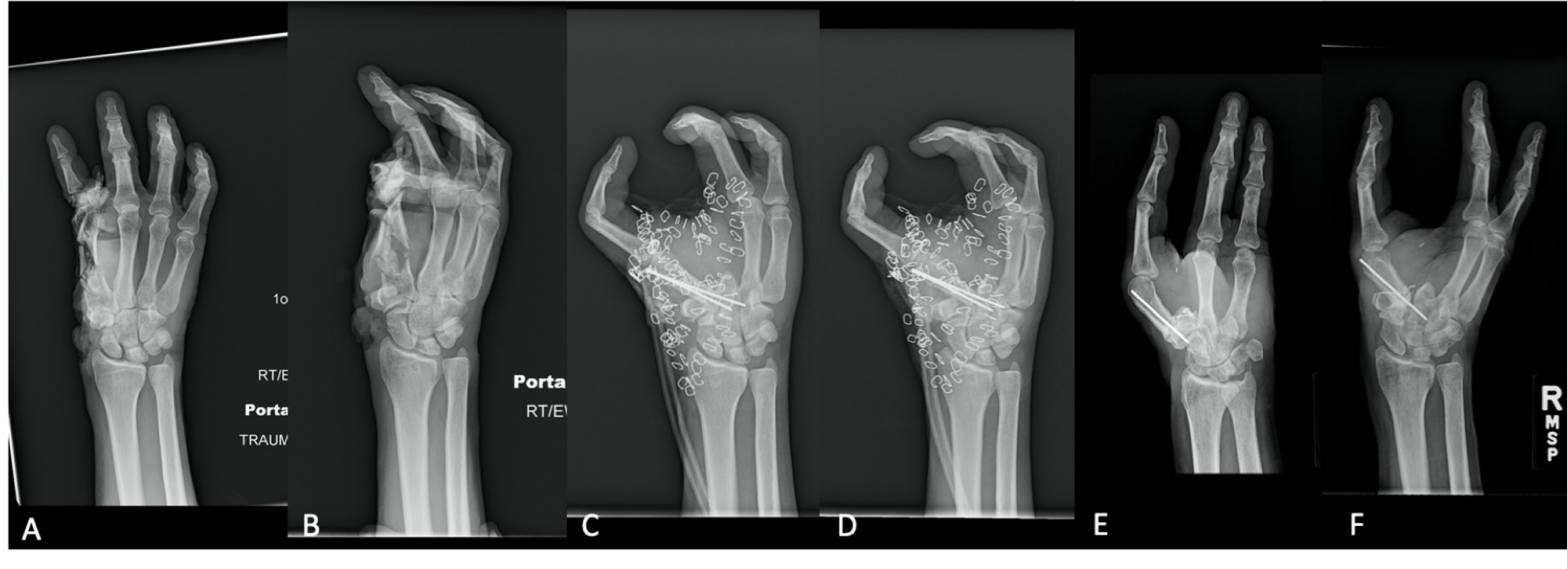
Radiograph images of patient's injury before and after pollicization (A) AP: Injury at presentation. (B) Oblique: Injury at presentation. (C) AP: After pollicization. (D) Oblique: After politicization. (E) AP: six-month follow-up. (F) Oblique: six-month follow-up. AP: anterior-posterior.
